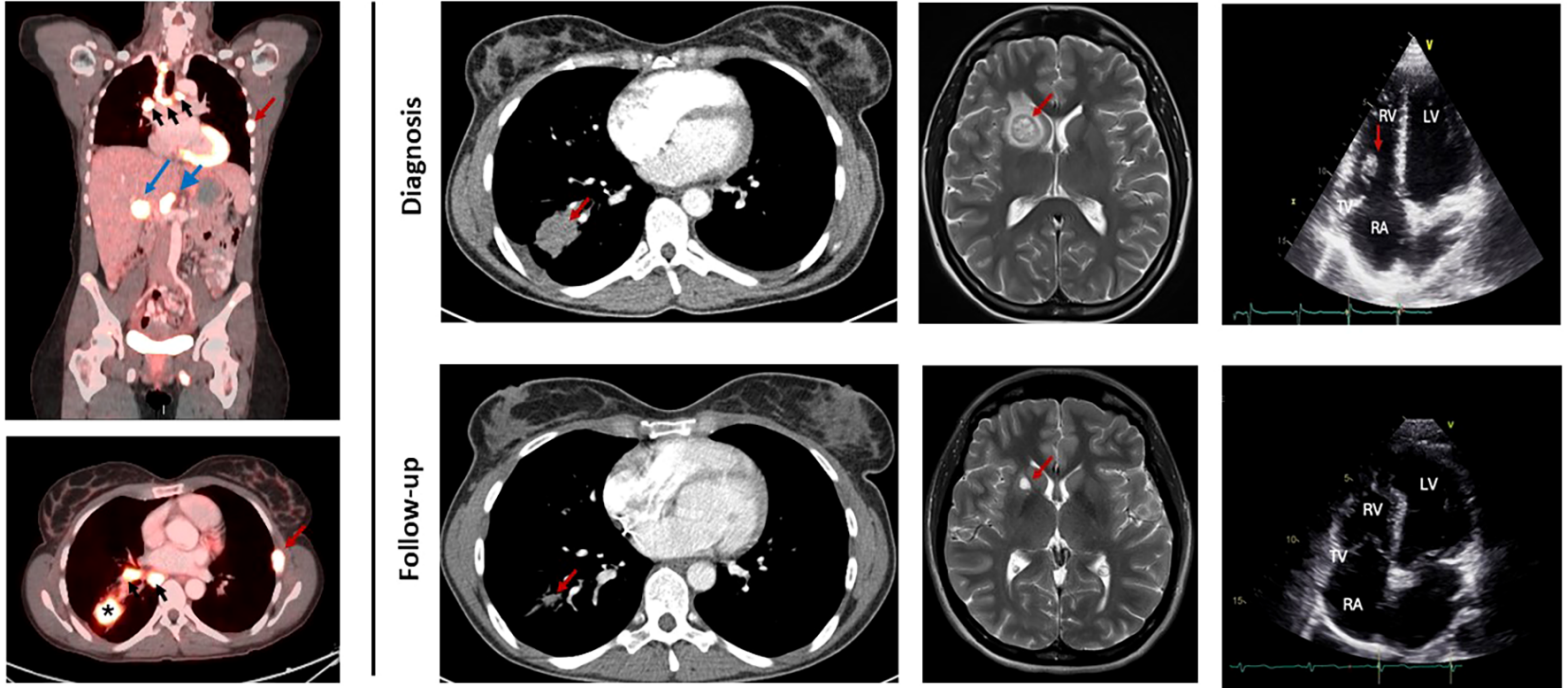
Oncological diagnosis and response to treatment. Left panel: PET-CT at diagnosis showing intense FDG-captation of the consolidation in the right lower lobe (black asterix), ipsilateral and contralateral mediastinal (black arrows) and hepatic hilar lymphadenopathies (blue short arrow), liver metastasis (blue long arrow) and multiple bone metastases (red arrow). Right panel: Treatment response contrast enhanced chest CT (left) showing volume reduction of primary tumor in the right lower lobe (red arrow) and brain MRI T2-weighted, contrast-enhanced image (middle) showing volume reduction of brain metastasis in the right lentiform nucleus (red arrow) after 1 year of treatment with Osimertinib. Transthoracic echocardiography, apical 4 chamber view (right) showing tricuspid valve vegetation at diagnosis (red arrow) and no residual vegetation after 4 months of treatment with Osimertinib. RA, right atrium. RV, right ventricle. LV, left ventricle. TV, tricuspid valve.
